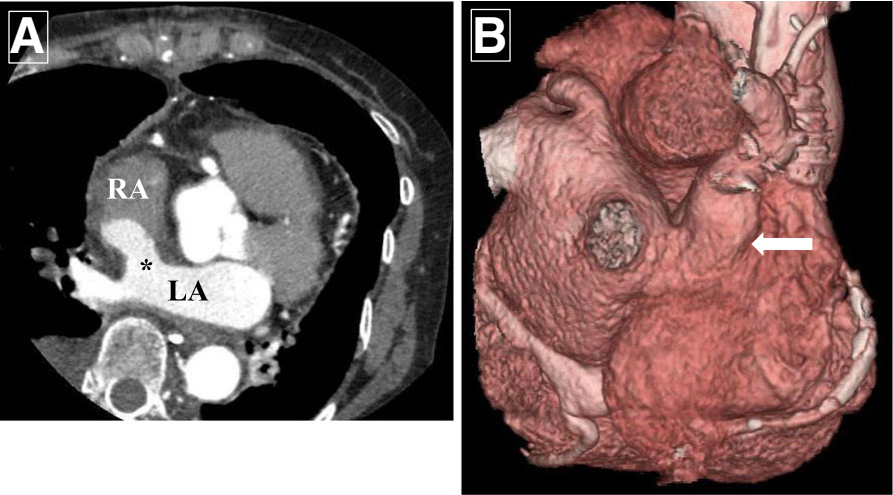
Postoperative computed tomography (CT) findings. a Left atrium (LA) and right atrium (RA). *Surgically created atrial septal defect. b Three-dimensional CT shows new pathway from right upper pulmonary vein to LA (*arrow*)
